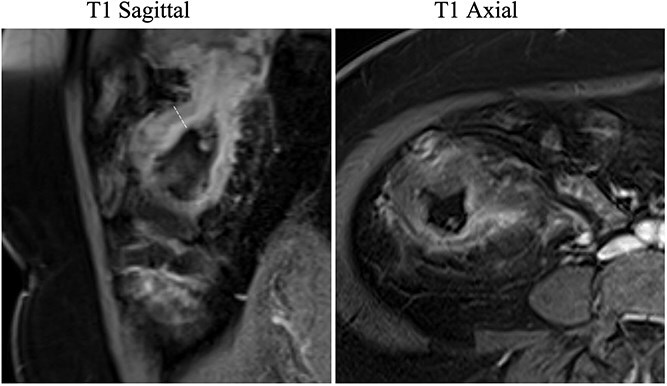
Pre-operative MRI of the abdomen and pelvis. There is an approximated 4–5 cm of decompressed colon/cecum with inner layer hyperenhancement and bowel wall thickening measuring up to 1.2 cm with involvement of the ileocecal junction and extension into the terminal ileum. There is no abnormal upstream dilation of the small bowel.
